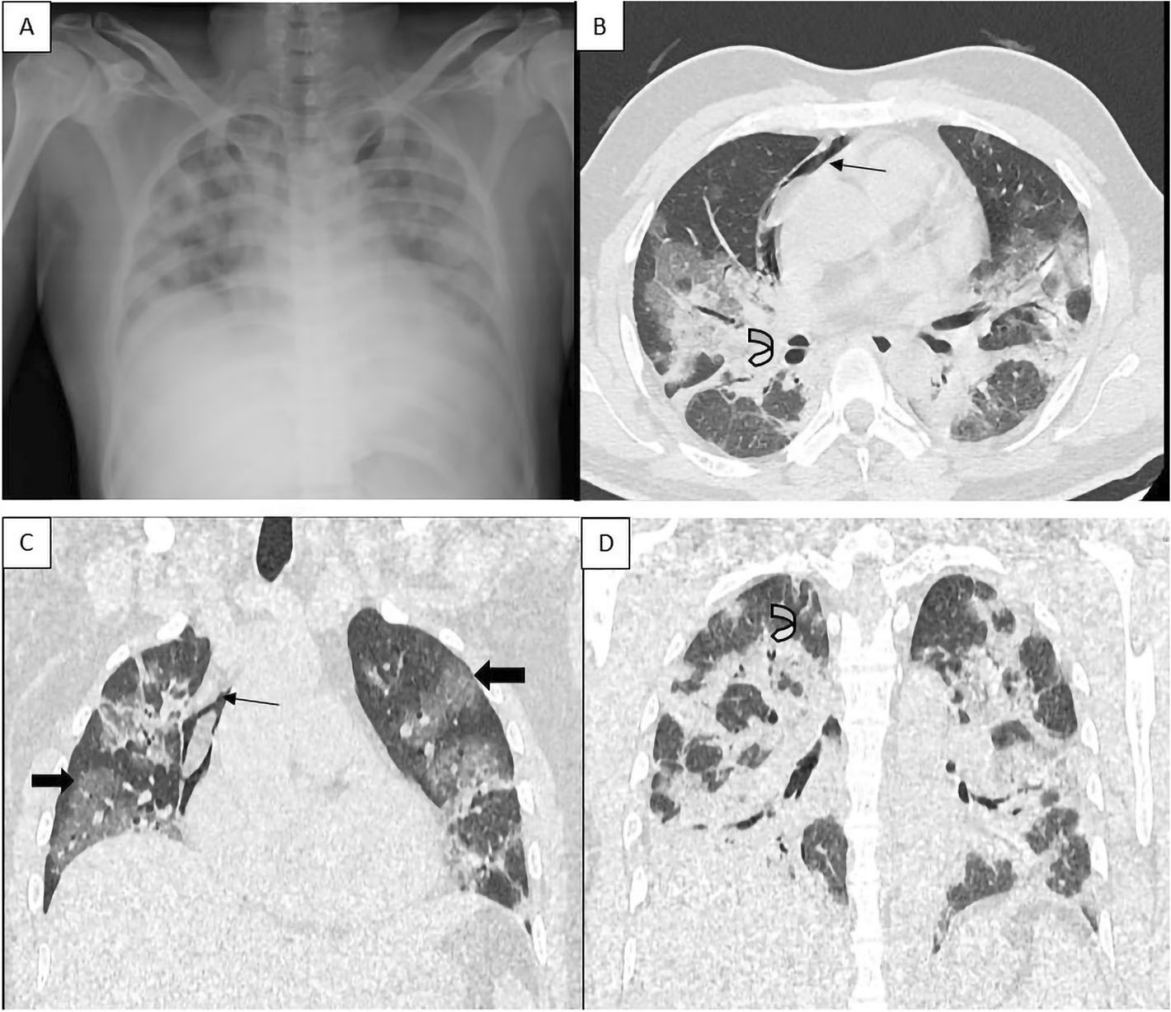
Chest x-ray (a) non-homogenous patchy opacities in bilateral lung fields suggestive of consolidation. Axial HRCT section (b), coronal sections (c, d) demonstrate the presence of air in the mediastinum (black arrow) along with the presence of diffuse ground-glass opacities (block arrow) and consolidation (curved arrow) with air bronchogram in the bilateral lung parenchyma
